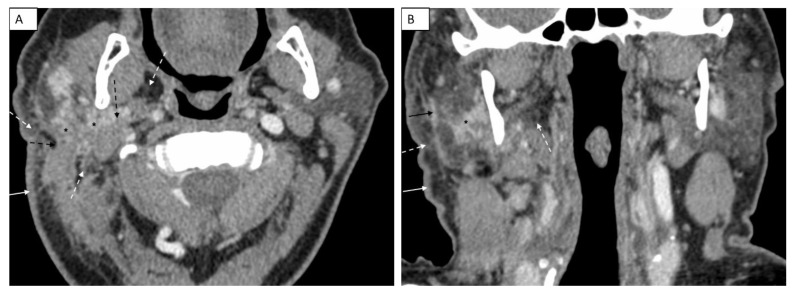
A follow-up post-contrast CT scan of the face 6 weeks later shows worsening skin thickening (white arrow), fat stranding and fat plane effacement (dashed white arrows), ill-defined margins with adjacent sternocleidomastoid and posterior belly digastric muscles with asymmetric enlargement (dashed black arrows) and increased ill-defined margins of the primary lesion in the right parotid gland (black asterisks) on the axial image (A). There is infiltration of parapharyngeal fat (dashed white arrow), increased ill-defined margins of the primary lesion in the right parotid gland (black asterisks) and fascial plane thickening (solid black arrow) on the coronal image (B).
